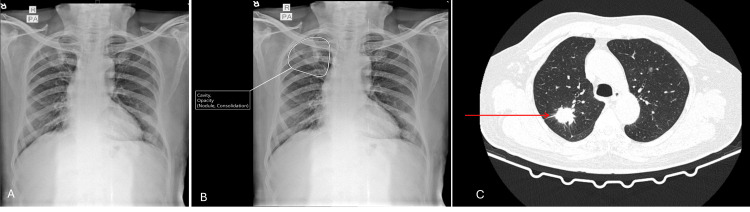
Chest X-ray and CT images of patient 5 A: Chest X-ray image without AI; B: Interpretation of chest X-ray with AI (suspicious nodules marked); C: Computed tomography (CT) image of the thorax (arrows show nodules)
